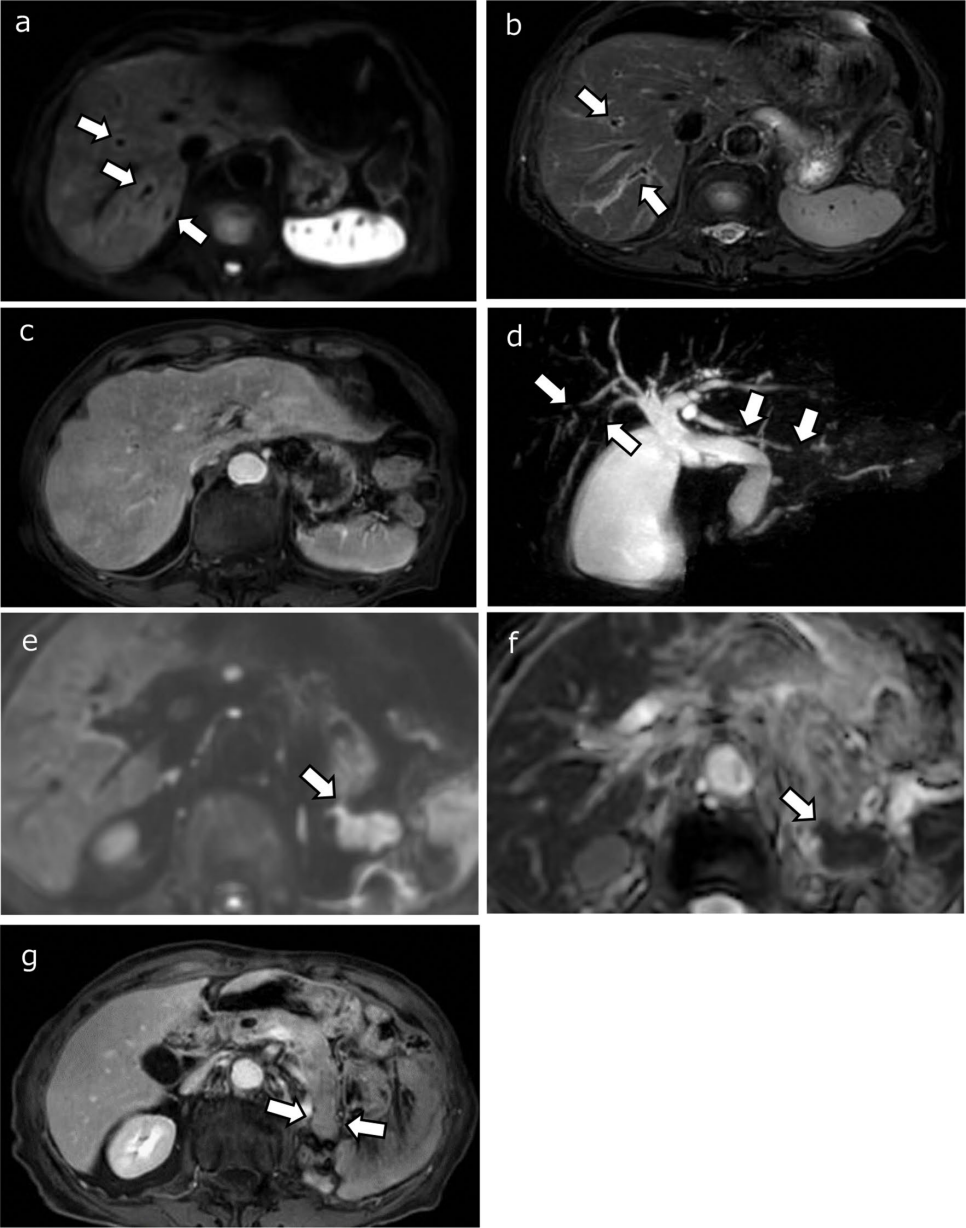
(a and e): Diffusion-weighted imaging (b = 800). (b): Fat-suppressed T2-weighted imaging. (c): Fat-suppressed contrast-enhanced T1-weighted imaging in the early phase (d): Magnetic resonance cholangiopancreatography (MRCP). (f): Apparent diffusion coefficient (ADC) map. (g): Fat-suppressed contrast-enhanced T1-weighted imaging in the portal venous phase. Faint high-signal areas are observed along Glisson’s sheath in the liver parenchyma on diffusion-weighted imaging and fat-suppressed T2-weighted imaging (a, b; arrows), suggesting edematous change with heterogeneous parenchymal enhancement in the early-phase image (c). Multiple luminal narrowings in the intrahepatic bile duct (d; arrows) and common bile duct dilatation are shown on MRCP. The enlarged pancreatic tail exhibits high signal intensity on diffusion-weighted imaging (e; arrow), with a reduced ADC value of 1.12 × 10^− 3^ mm^2^/s and decreased enhancement on fat-suppressed contrast-enhanced T1-weighted imaging (f; arrows)
